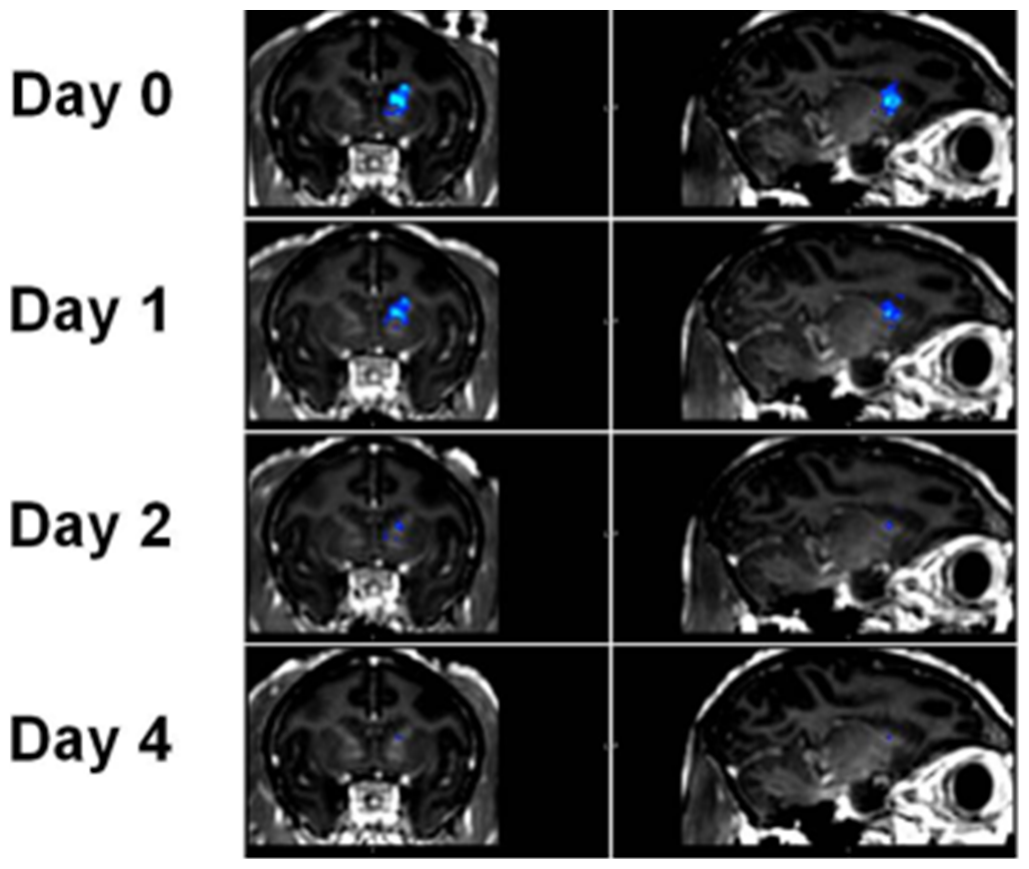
Coronal (left row) and sagittal (right row) T1-weighted MR slices showing the evolution of the BBB opening volume along time. The area with contrast agent diffusion is overlaid in blue. The BBB is restored between day 2 and 4.
